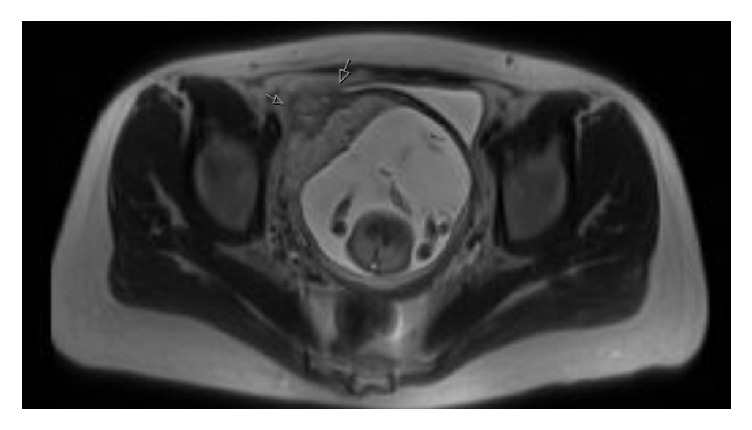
Cross-sectional MRI showing intrauterine pregnancy and CSP with suggestion of placental invasion to the bladder.
